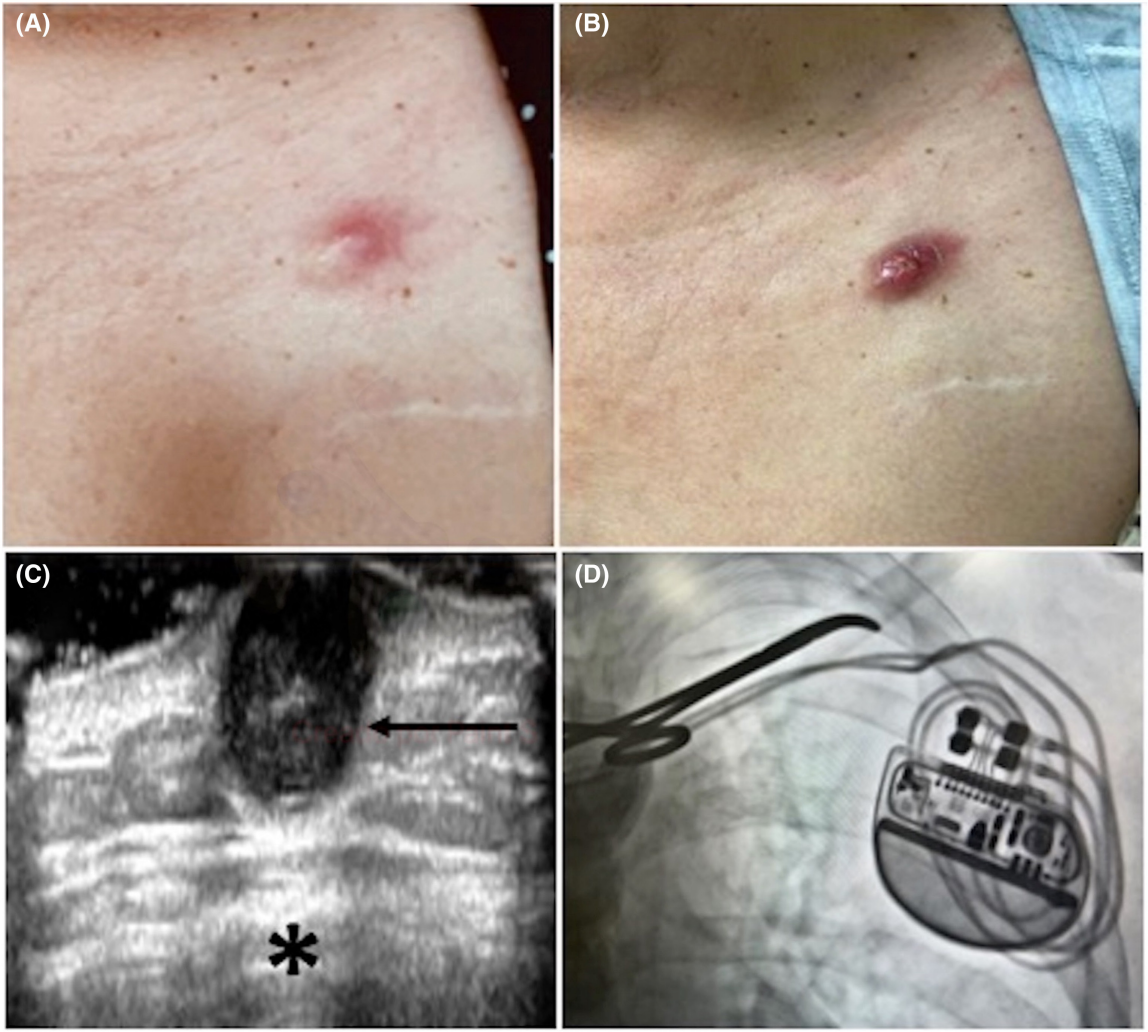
(A) A swollen, erythematous, and painful skin lesion at the upper part of the pacemaker pocket. (B) The lesion changed to a violaceous color and increased in size along with a superficial ulceration and fluid discharge. (C) An ultrasound scan of the skin lesion showed a hypoechoic and heterogeneous nodule with well‐defined borders located in the dermis and subcutaneous tissue (arrow). There was no compromise of deeper tissue and pacemaker pocket (asterisk). (D) Fluoroscopy showed that the skin lesion was over the upper part of the pocket where the leads run (under the Kelly forceps).
